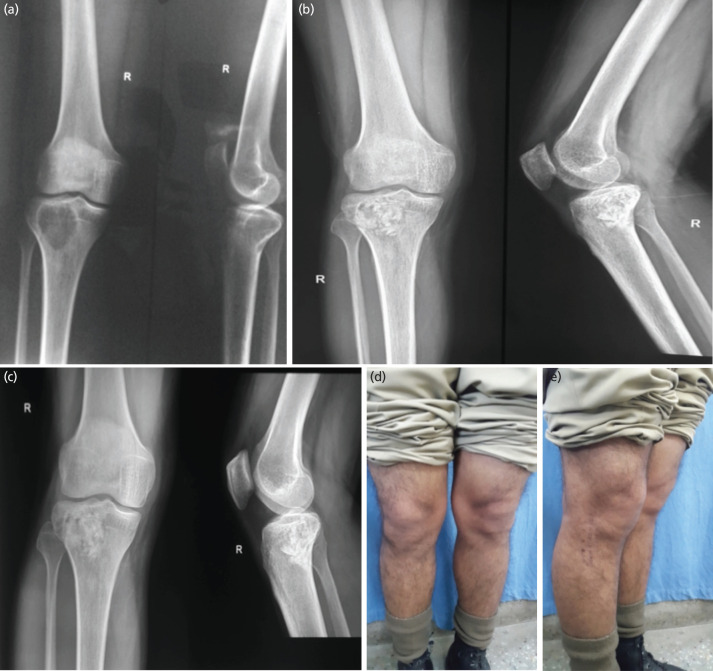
A 32-year-old male with Campanacci grade I GCT of the right proximal tibia operated by curettage and bone grafting (CBG). (a) A pre-operative radiograph (AP/Lateral) of the right knee with proximal tibia showing lytic lesion with a clear surrounding margin of bone present, suggestive of Grade I GCT of the right proximal tibia. (b) Immediate post-operative radiograph of a patient treated with extensive curettage and bone grafting from the contralateral iliac crest. (c) Radiograph at the final two-year follow-up showing a well-healed lesion with no signs of GCT recurrence. (d and e) Clinical photographs of the patient's capabilities at the two-year follow-up showing a full range of motion and complete ability to squat.
